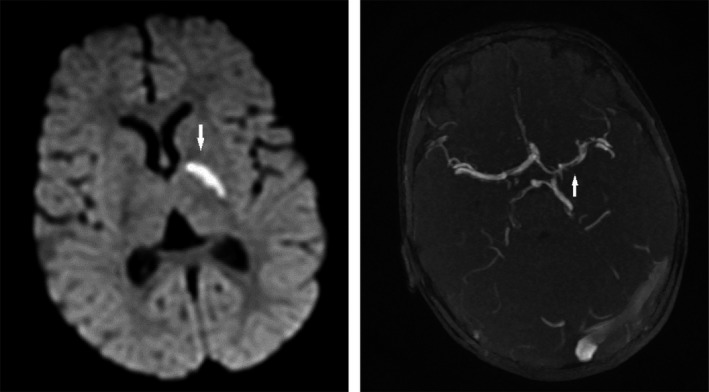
MRI brain: on the left, ischemic injury in the left capsulo‐thalamic region (T2‐weighted image, axial plane), while on the right, the angio‐MR image showing a thinner and irregular aspect of the left arteria cerebri media
